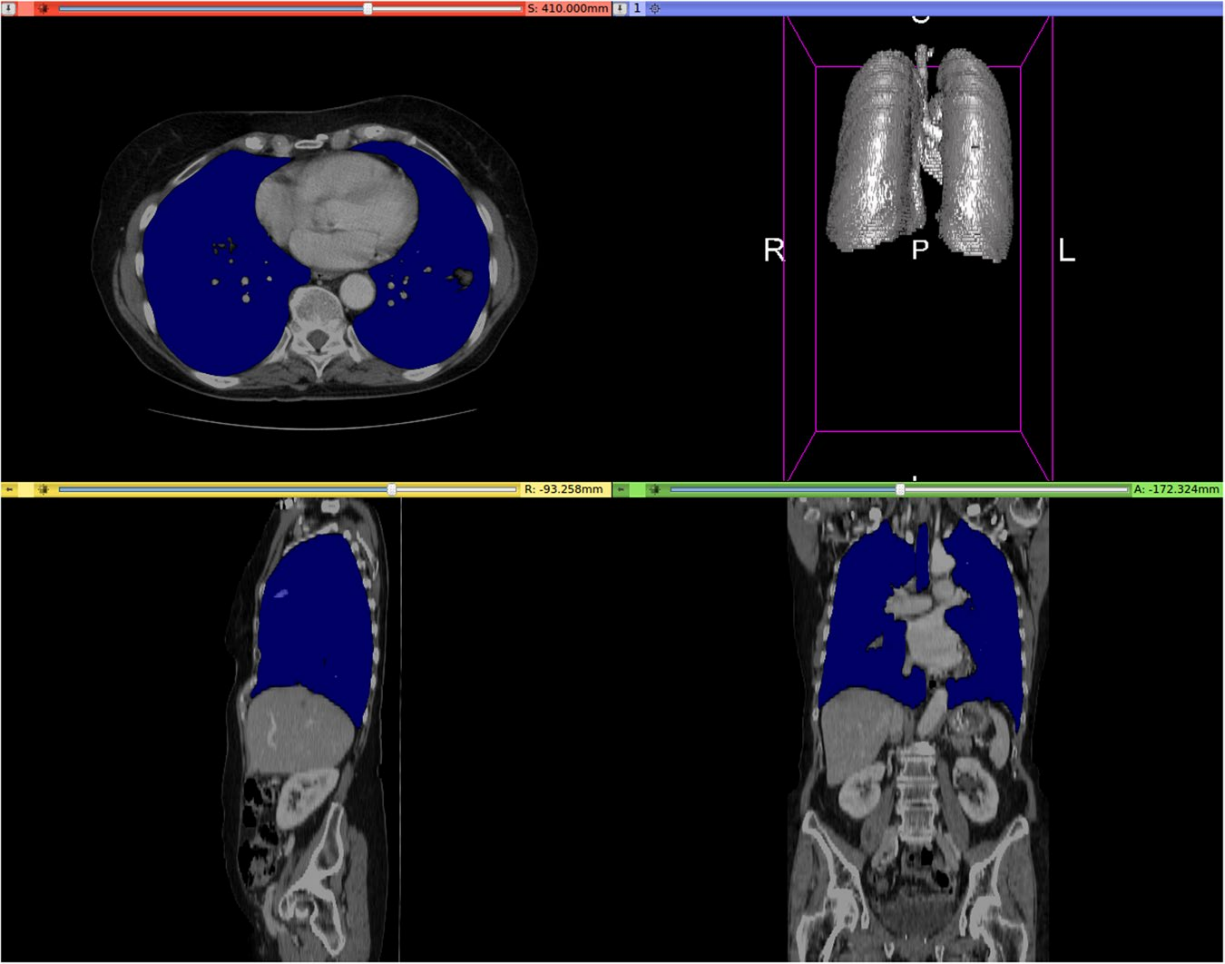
Example of CT lung detection and segmentation by image morphology. Lung mask overlaid in blue. Rendered by 3D Slicer^24^.
